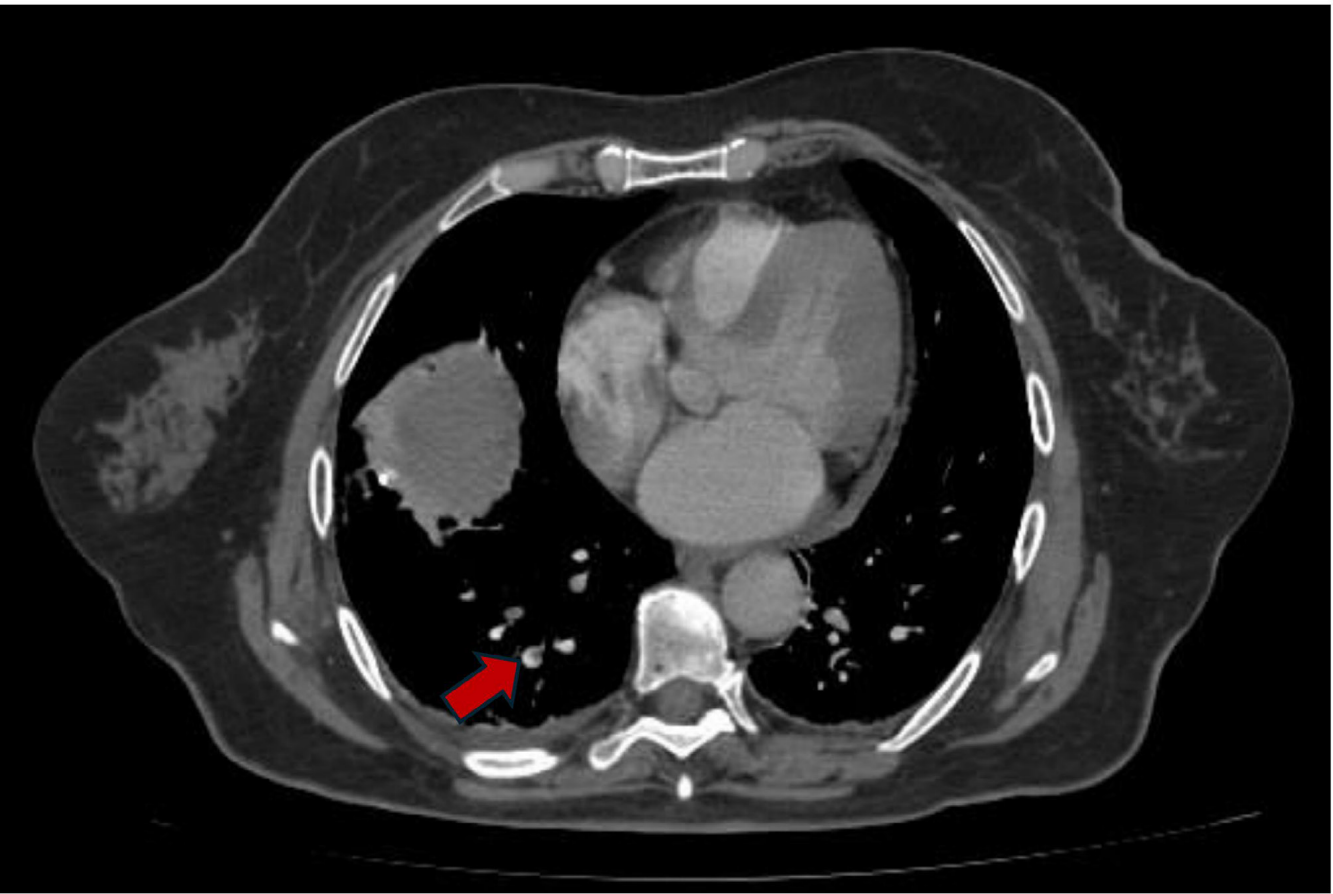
CT pulmonary angiography showed thrombotic appositions in the lower right lobar pulmonary artery (red flag).
